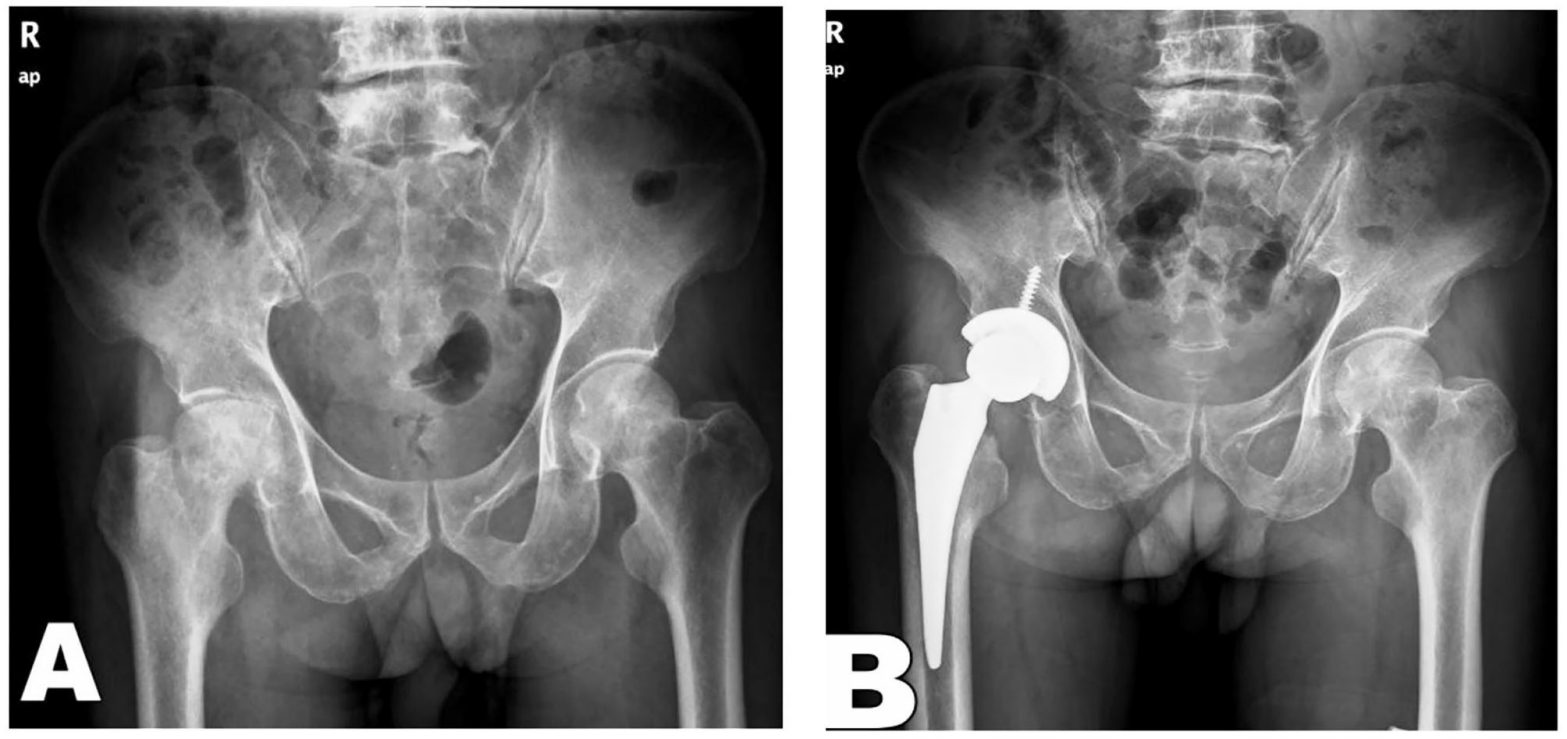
Preoperative X-rays indicated Ficat stage IV of hip avascular necrosis of the right femoral head and subjected to DAA for total hip arthroplasty. (A) Preoperative orthographic X-ray; (B) Postoperative orthographic X-ray.
